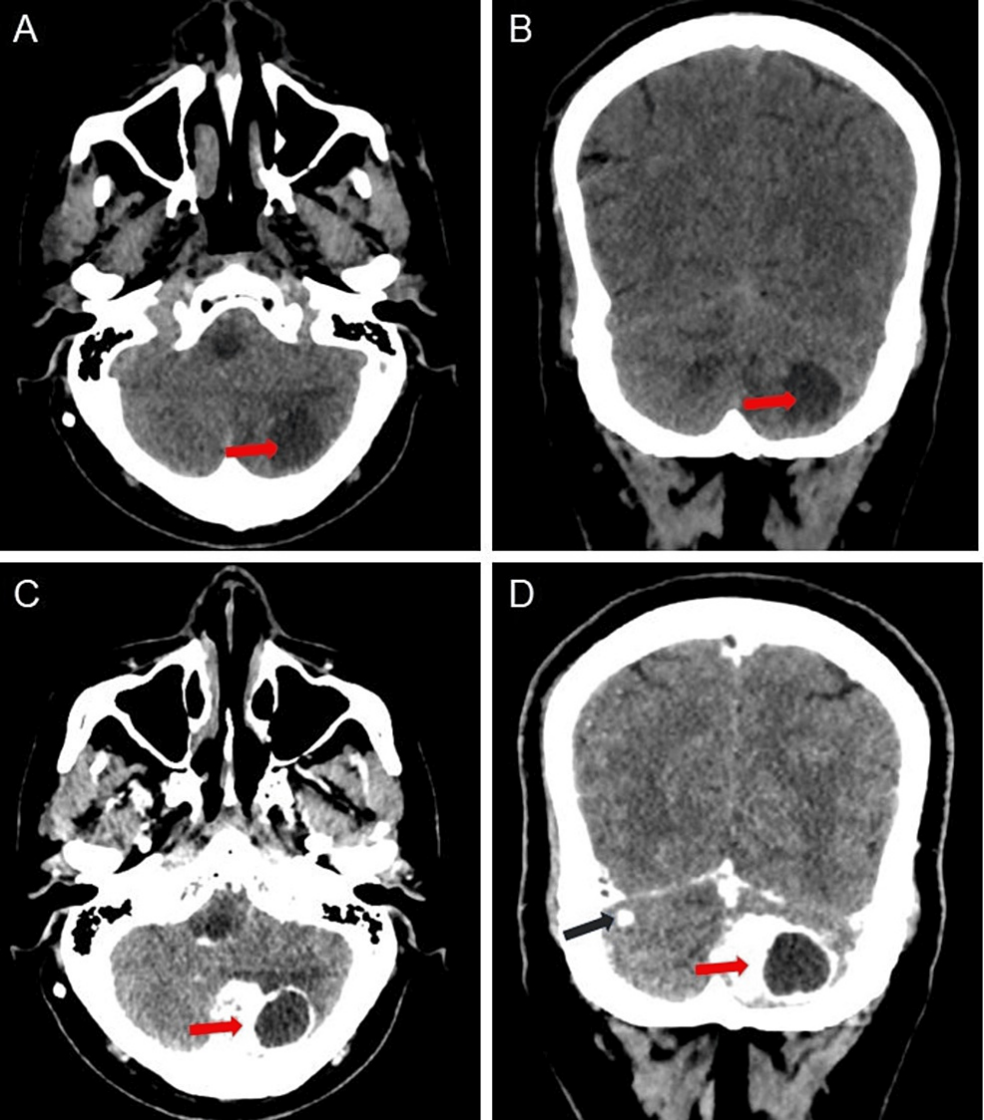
Axial and coronal reformatted non-contrast and post-contrast computed tomography images of the brain (A)-(B): Axial and coronal reformatted non-contrast computed tomography images show a solid cystic lesion in the left posterior cerebellar hemisphere (red arrows) (C)-(D): Axial and coronal reformatted post-contrast images show avid post-contrast enhancement of solid mural nodules (red arrows), and a small enhancing lesion is seen in the right cerebellar hemisphere (black arrow)
